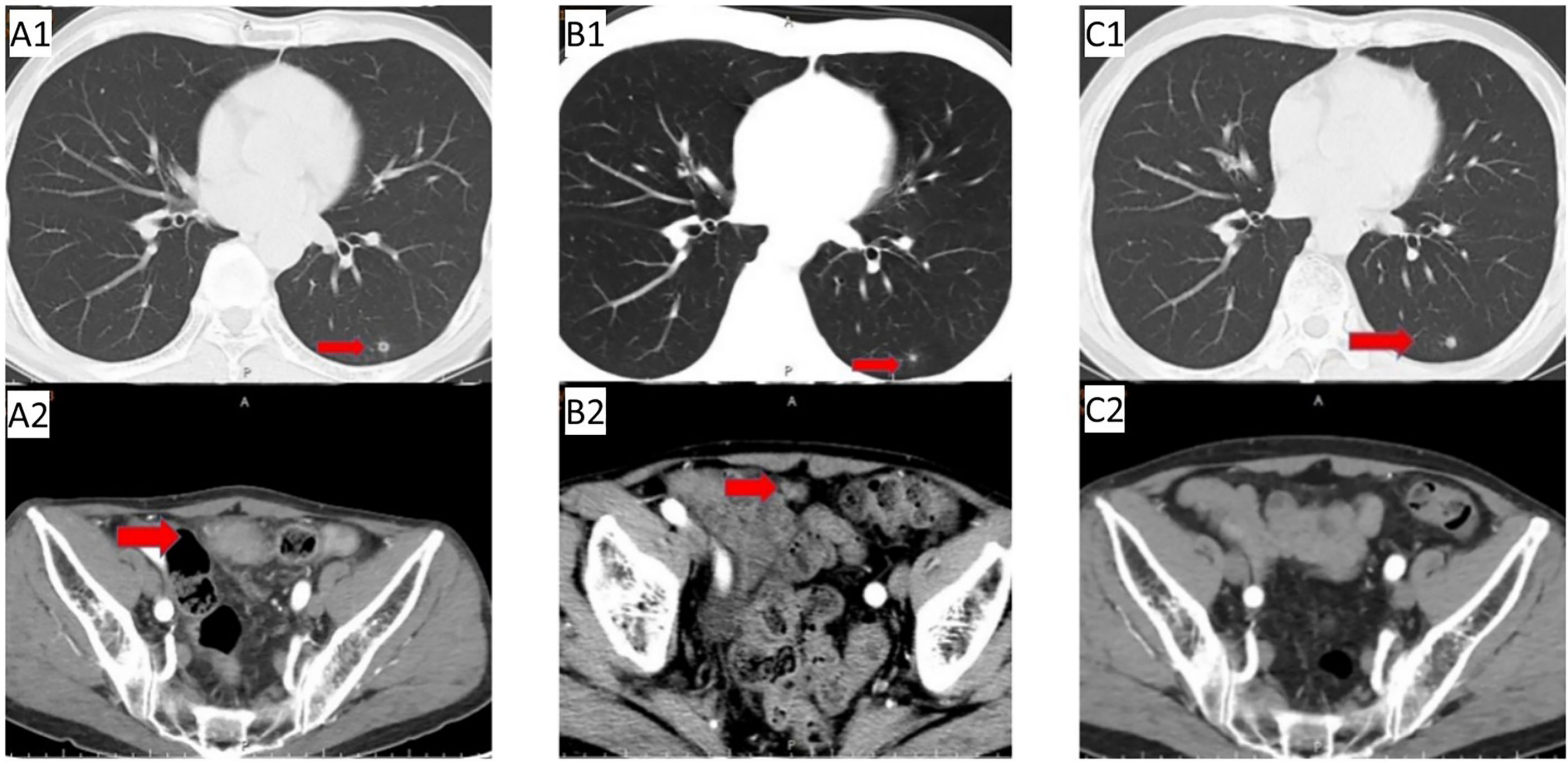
First-line treatment: (A1/A2) Baseline CT before first-line treatment; (B1/B2) CT after 2 cycles showed left lung nodules similar to before, pelvic metastases were significantly reduced; (C1/C2) follow-up CT on May 29, 2019 showed slightly enlarged left lung nodules, no pelvic metastases were observed.
